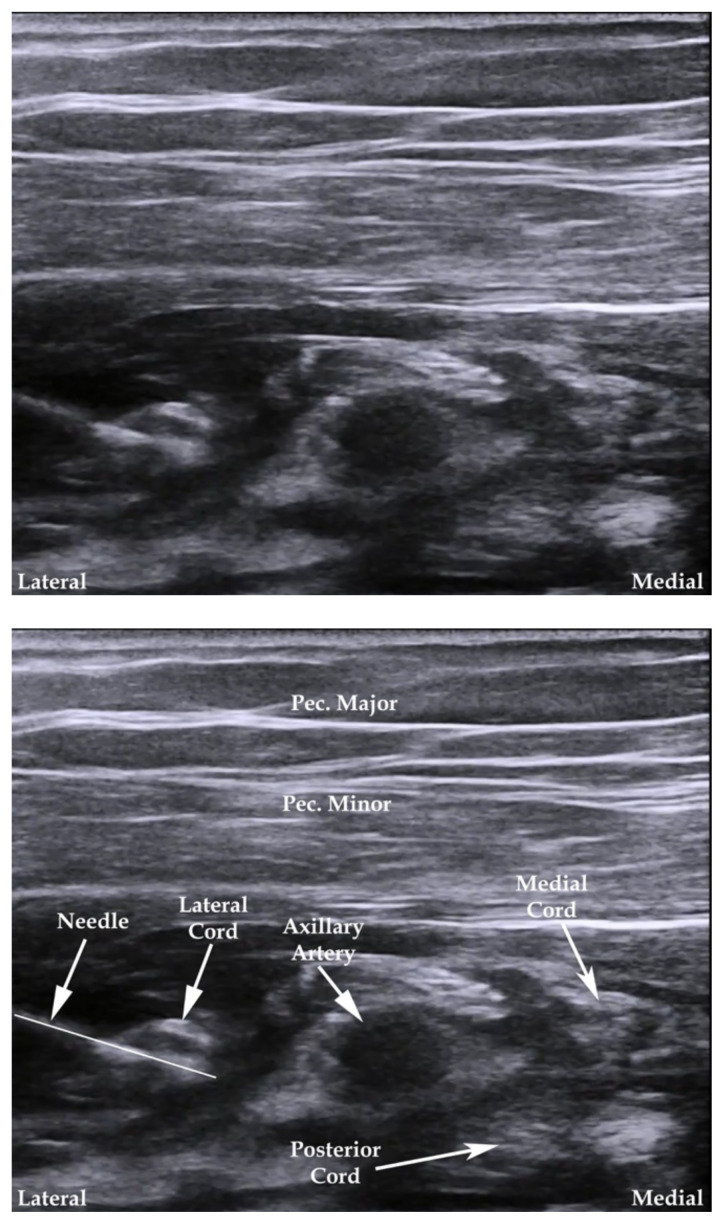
Ultrasound-guided infraclavicular brachial plexus nerve block. Modified from source.^14^
